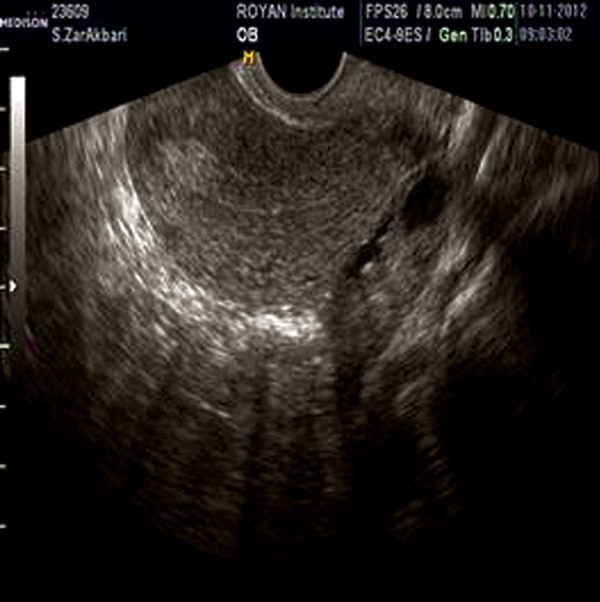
Sagital transvaginal sonogram showing anteverted uterus
